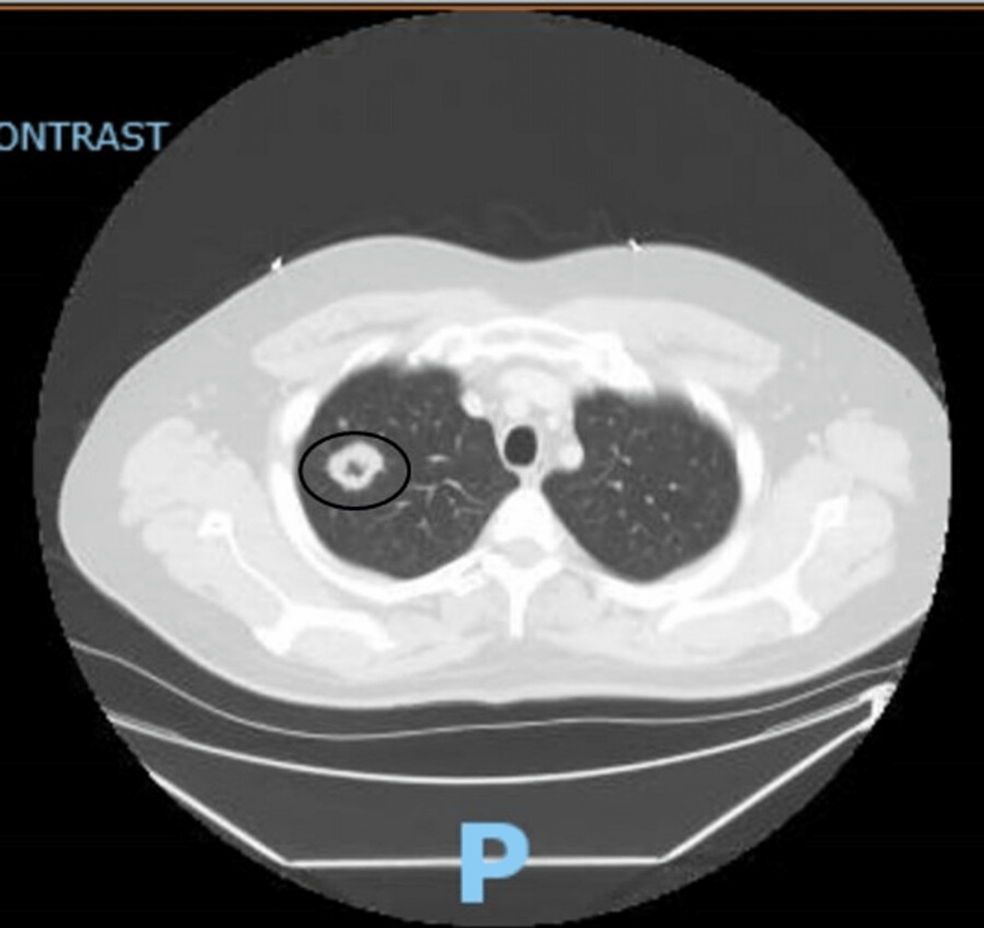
CT of the chest with a cavitary chest lesion.
